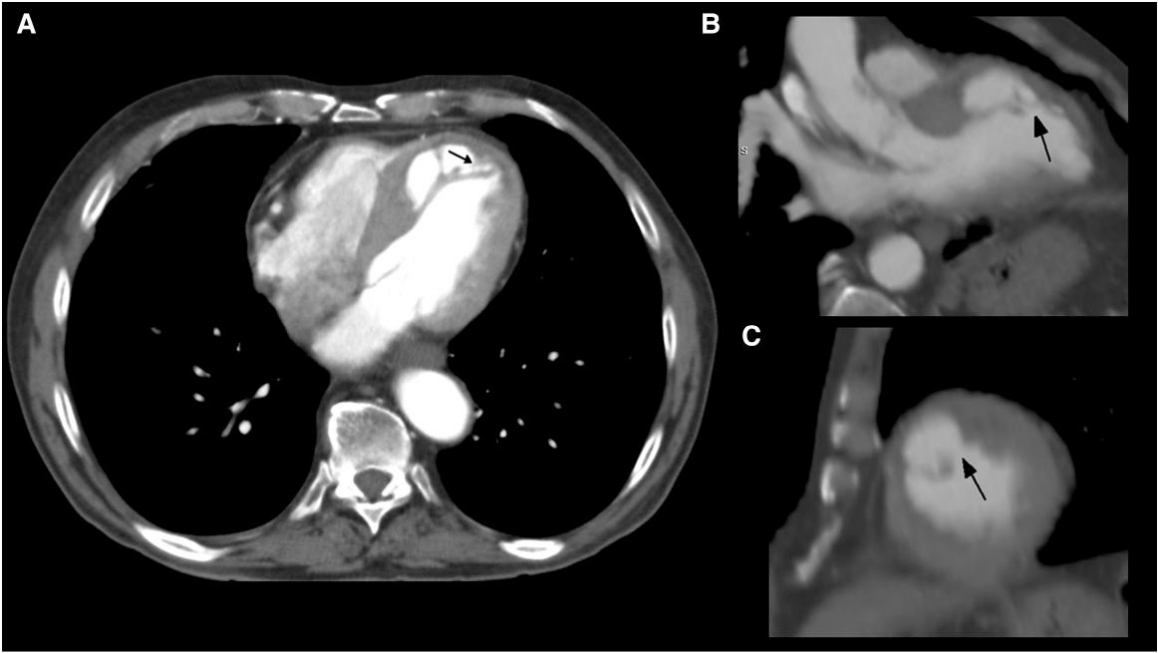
Cardiac CT images in Patient 5. A4C (*A*), A3C (*B*), and SA (*C*) views after multiplanar reconstruction. We note an intramyocardial dissecting cavity communicating with the LV through a narrow neck (arrows).
